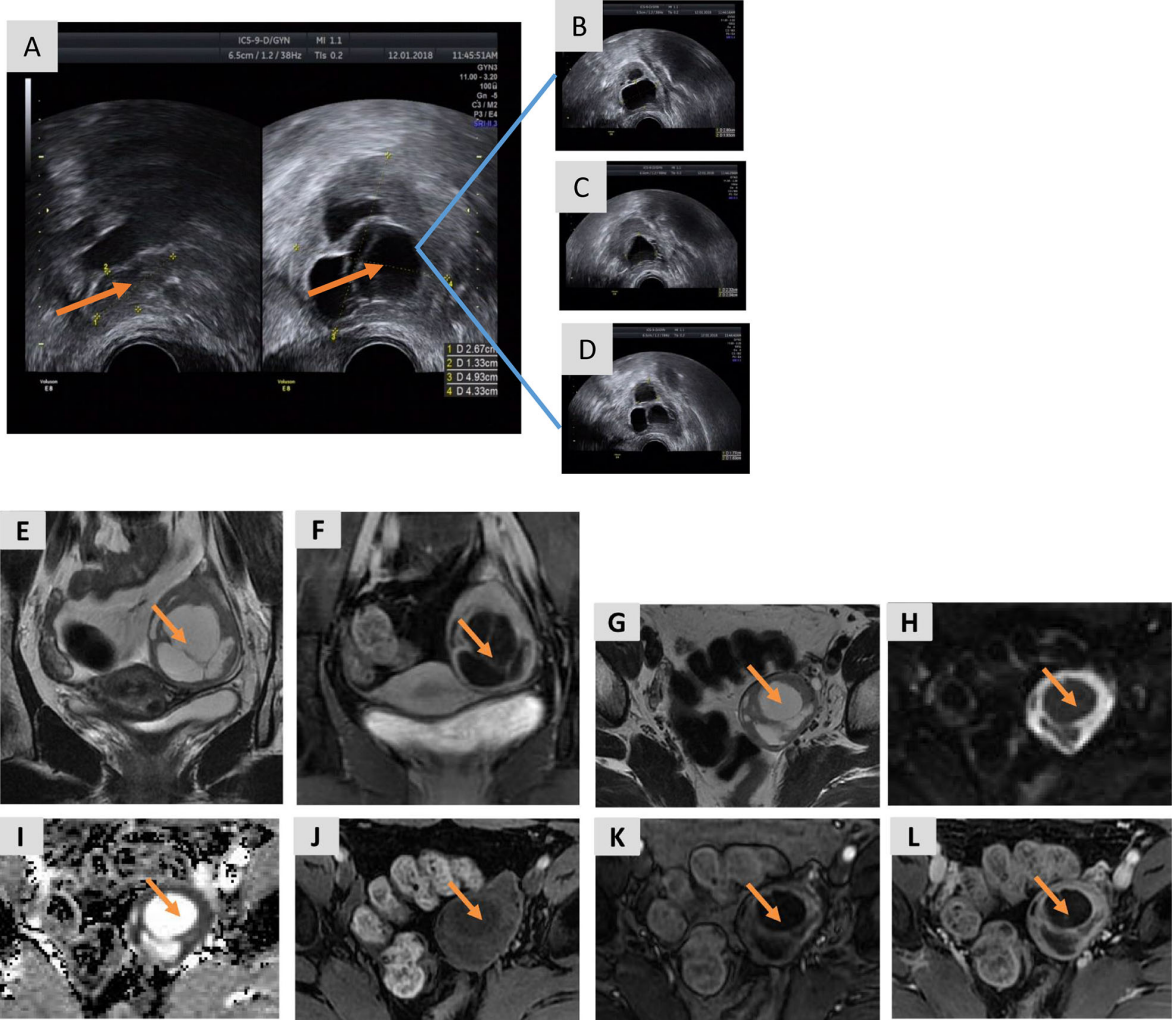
Transvaginal ultrasound images and MRI of the pelvis prior to ovarian cystectomy. (A) The right ovary is normal, measuring 2.7 × 1.3 cm with 2–3 antral follicles, while the left ovary is enlarged, measuring 4.9 × 4.3 cm with 2–3 antral follicles. (B–D) The three cysts in the left ovary measure 2.8 × 1.9 cm (B), 2.3 × 2.0 cm (C), and 1.8 × 1.6 cm (D). (E) A coronal T2-weighted image shows a large, multicystic mass in the left ovary with a slightly hyperintense, solid component of the septa and a thickened wall. (F) A coronal T1-weighted image with fat saturation of the delayed phase obtained after gadolinium administration shows marked enhancement of the solid components of the tumor. (G) An axial T2-weighted image shows a large, multicystic mass of the left ovary with septa and a thickened wall. (H, I) DWI (b=1,000) (Panel H) and the ADC map (I) show restricted diffusion of the solid components. (J) An axial T1-weighted image with fat saturation before enhancement shows hypo-intensity of the lesion. (K) An axial T1-weighted image of the arterial phase obtained after gadolinium administration shows marked early enhancement of the solid component of the tumor. (L) An axial T1-weighted image with fat saturation of the delayed phase obtained after gadolinium administration shows persistent enhancement of the solid component of the tumor.
